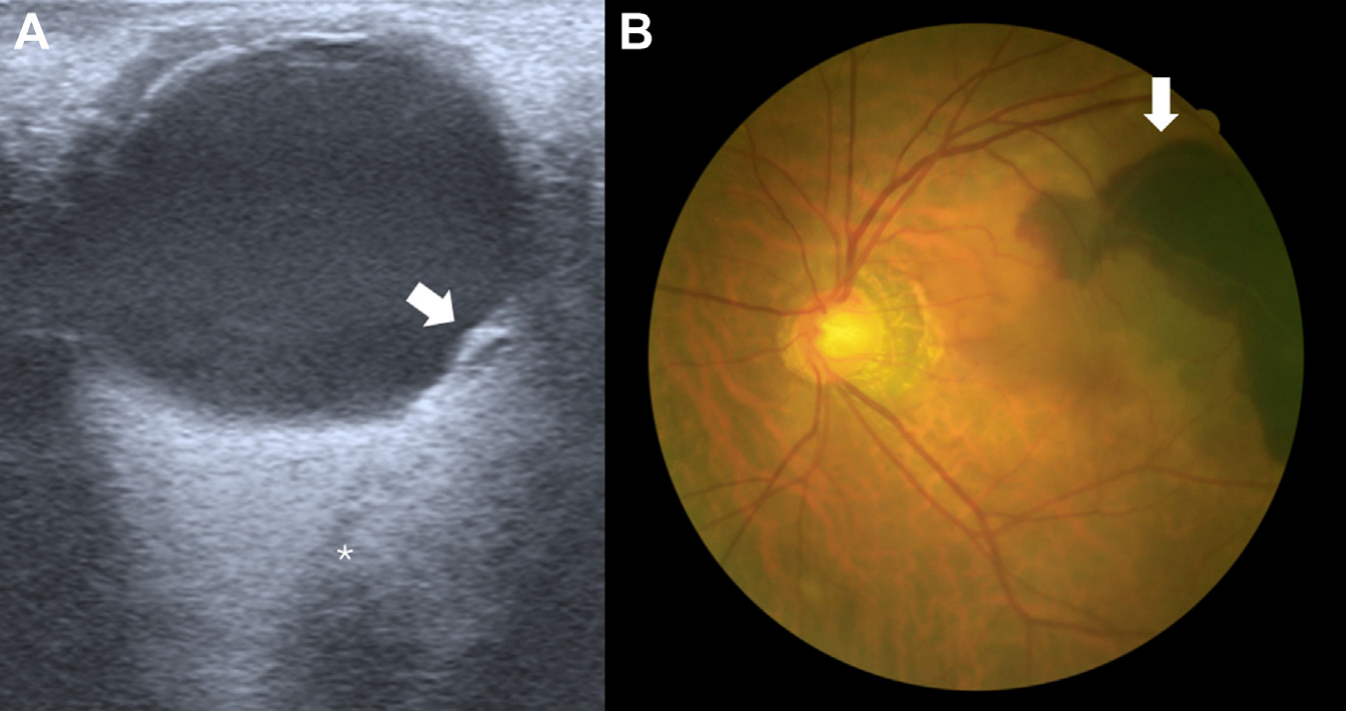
(A) The ocular ultrasound showed a bulging retina (arrow) with a mixed echogenic material posterior the lesion. ∗Indicates the optic nerve. (B) The fundus photography showed an irregularly shaped area of black discoloration (arrow) beneath the retina.
